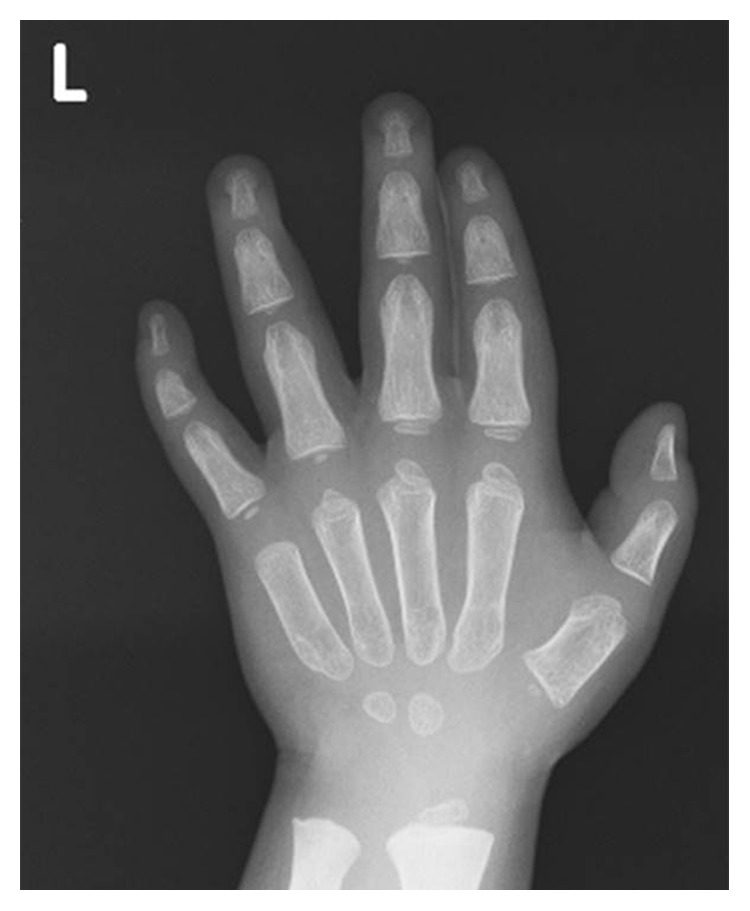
X-ray image of the fingers revealing sharp metacarpals.
